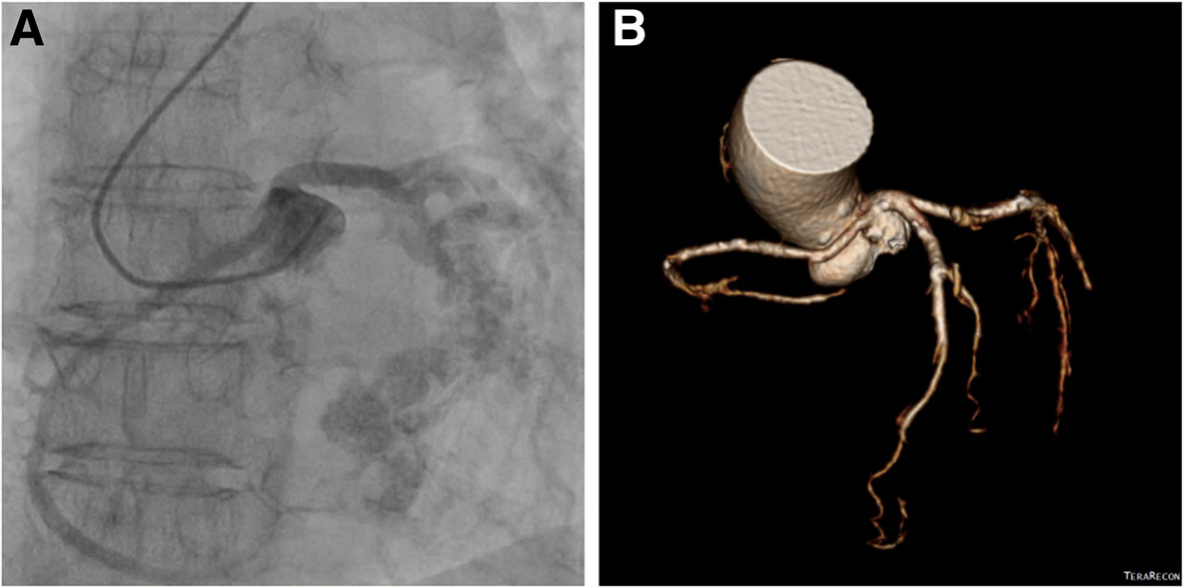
Preoperative imaging. a Coronary angiography images. b Computed tomography coronary angiography images. A type LII-B single coronary artery was observed according to Lipton’s classification
